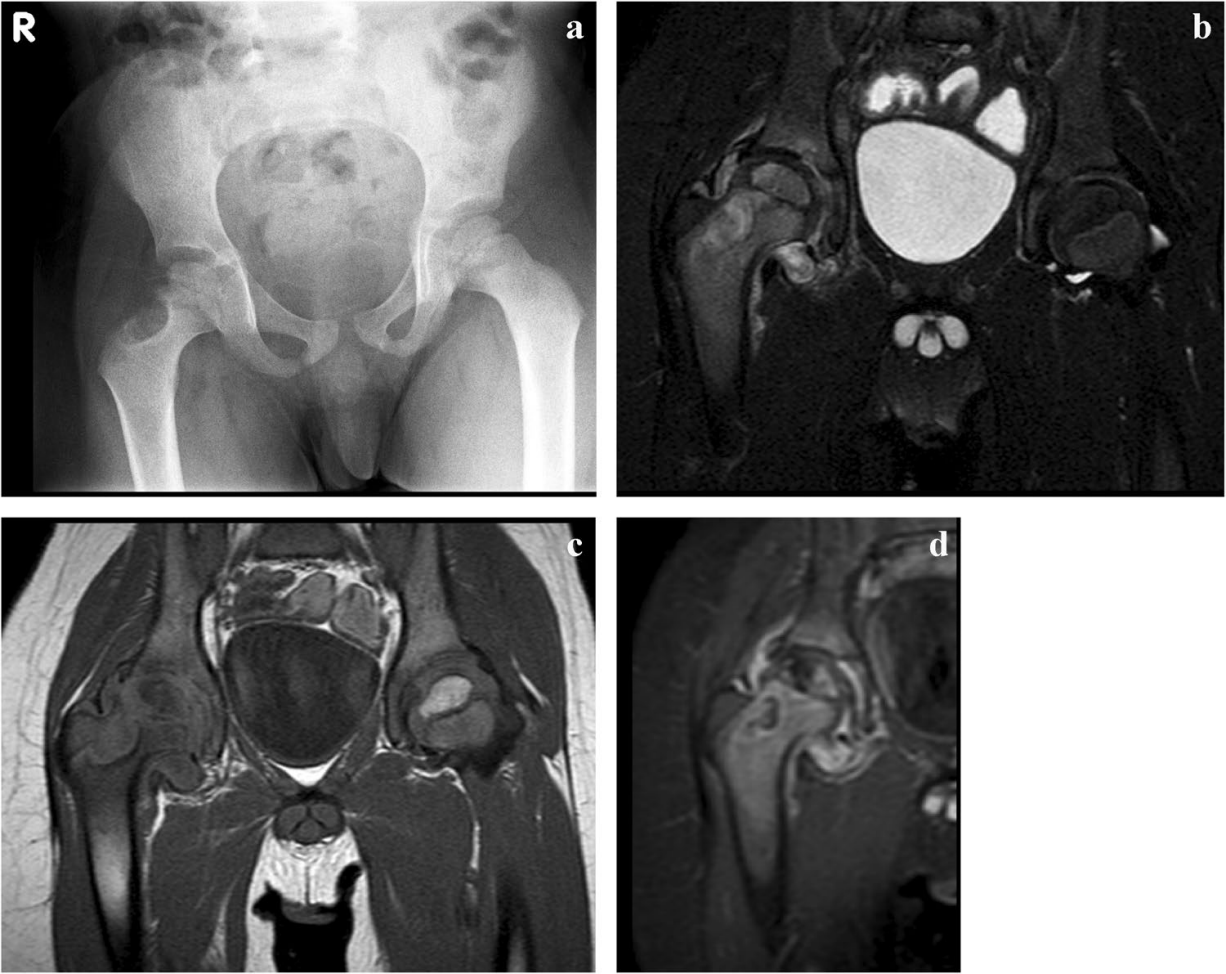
Cystic tuberculous (TB) osteomyelitis in a 7-year-old boy who had been limping since a minor fall 3 months previously. a An anteroposterior pelvic radiograph shows a well-defined eccentrically located cystic lesion of the lateral right femoral neck with cortical irregularity of the adjacent metaphysis. The femoral head is osteopaenic. b-d Coronal magnetic resonance images. T2 with fat suppression (b) and T1 (c) confirm involvement of the metaphysis and epiphysis and that the process crosses the joint to involve the superolateral acetabulum with a small associated effusion. There is heterogeneous enhancement of the bone and diffuse synovial enhancement post-contrast (d). Bone biopsy–confirmed tuberculosis
